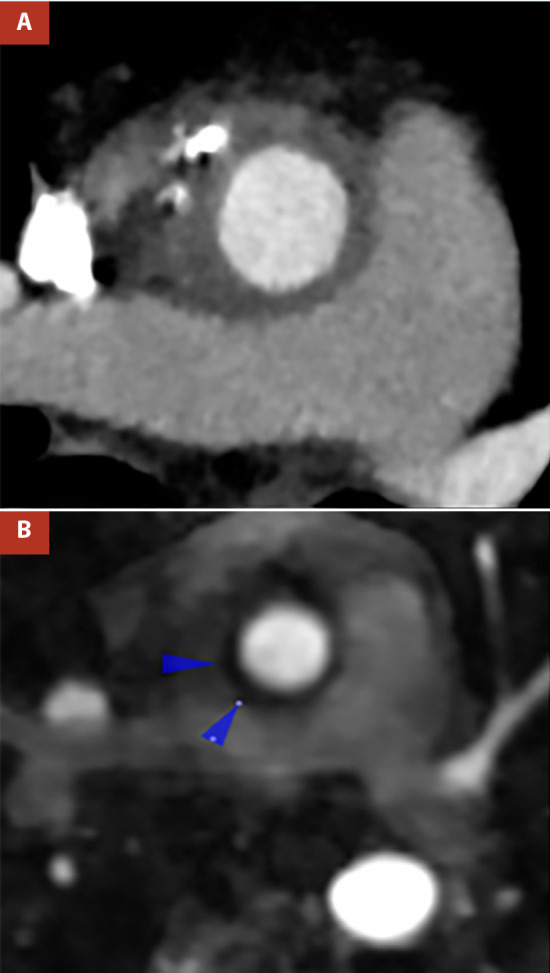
(A) Contrast Computed tomography angiography after corticosteroid therapy revealed decreased thickening of the aortic wall of 8.5 cm and attenuation of 50UH. (B) Post-treatment post-contrast resonance angiography revealed a decrease in aortic wall thickness (8mm) with hypointense signal. (blue arrow)
